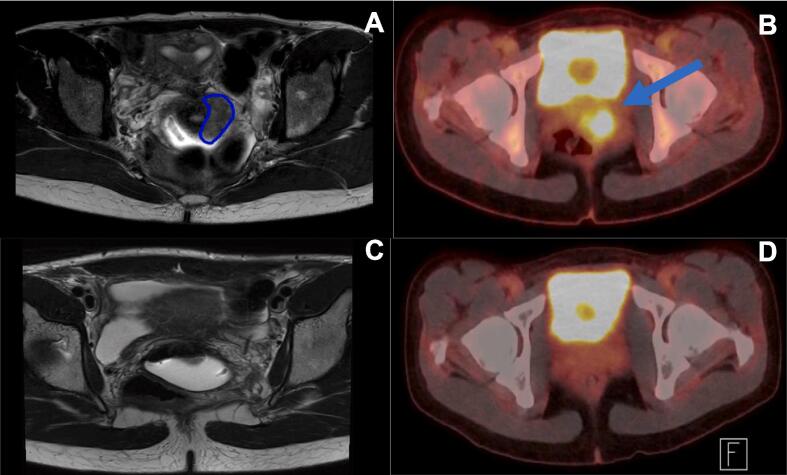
Radiologicalcompleteresponse after immuno-chemo-radiotherapy. A-B Diagnostic MRI showing a lesion of the cervix extending to the left parametrium, hypermetabolic on the PET-CT (SUV 8.8). C-D. Complete morphological and metabolic response 3 months after the end of treatment on the MRI (Fig. 1c) and PET-CT (Fig. 1d) respectively.
